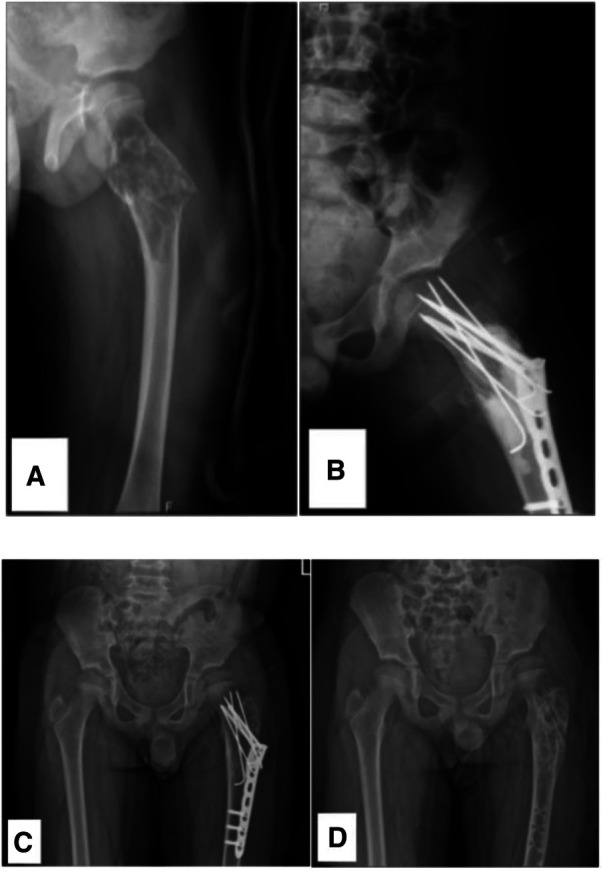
A 7.8-year-old male diagnosed with a SBC in the proximal left femur with an associated fracture. (A) Preoperative radiograph. (B) Radiograph taken 3 days post-biopsy, curettage, LCP-PHP, K-Wires procedure, and bone grafting. (C, D) Radiographs at 1.8 years post-surgery and following the removal of the internal fixation. As time progresses and the patient's femoral neck undergoes growth and development, the K-wire initially placed across the epiphyseal plate now appears to be positioned outside it.
